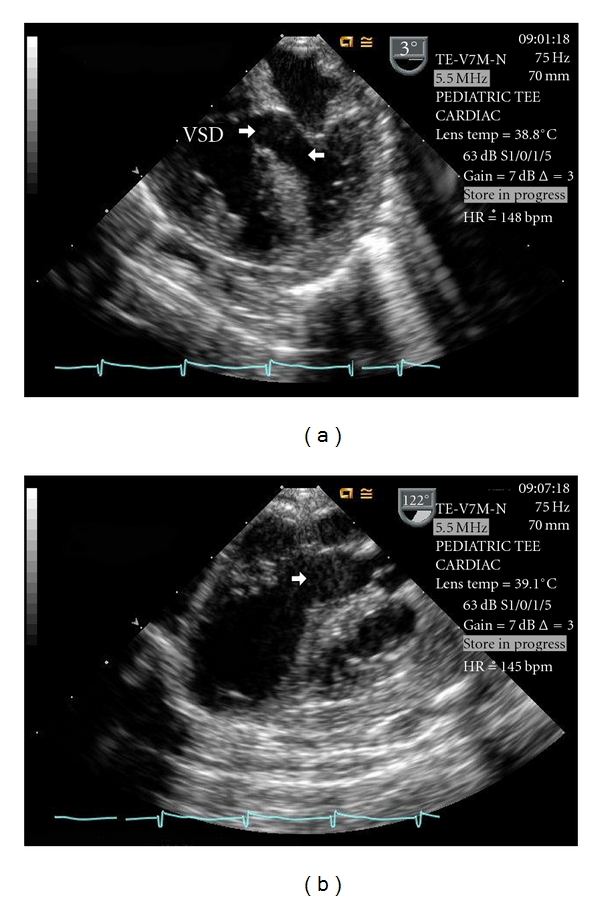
Intraoperative TEE demonstrating a patent LVOT (unlabeled arrow) and the large membranous VSD. Key: LVOT—left ventricular outflow tract, TEE—transesophageal echocardiogram, VSD—ventricular septal defect.
